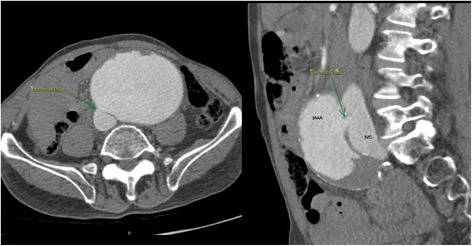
Abdominal CT with IV contrast demonstrating a large infrarenal AAA ruptured in the inferior vena cava in axial and sagittal view
